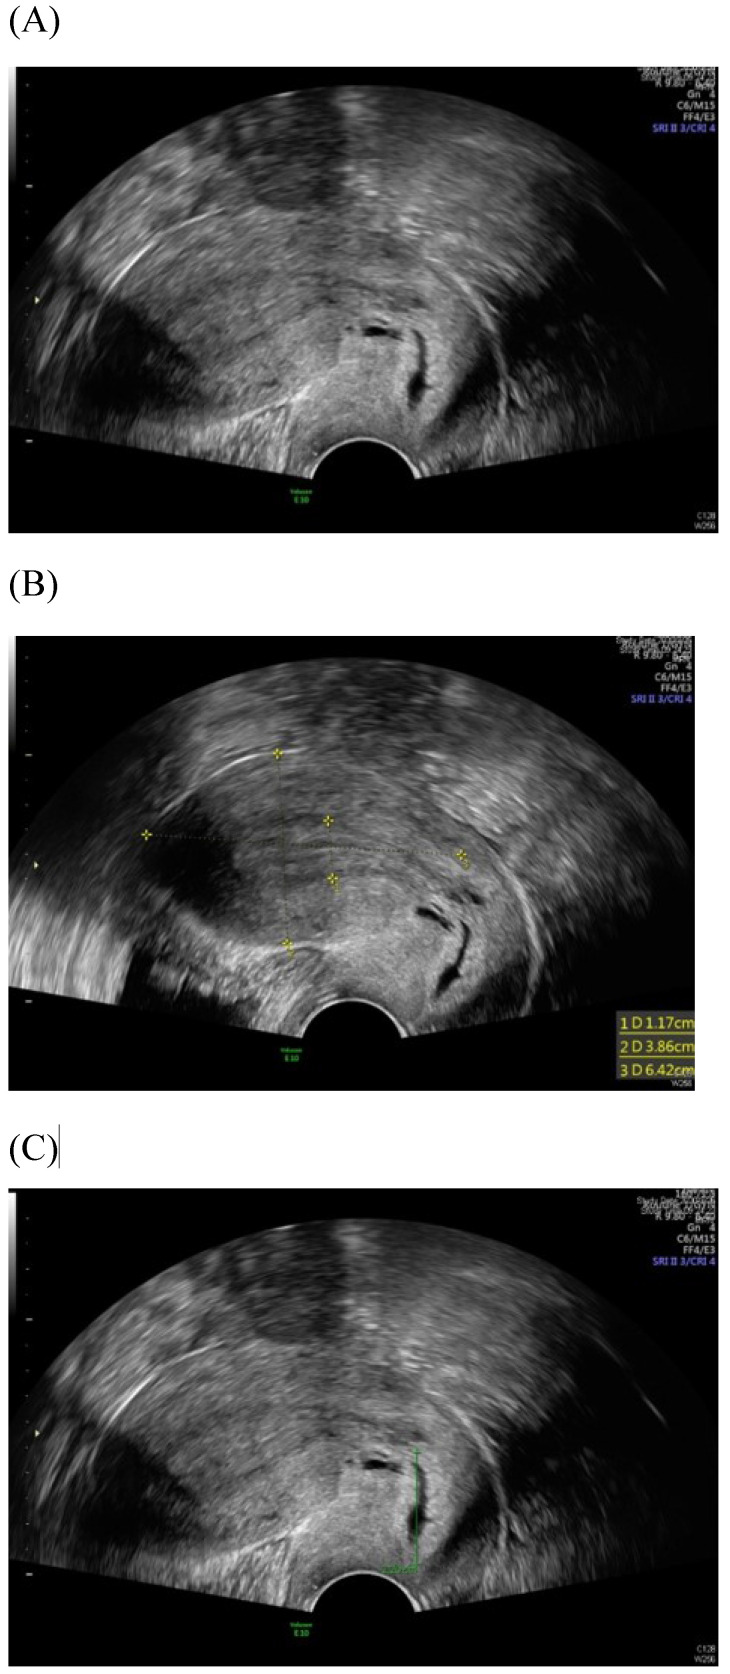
Transvaginal ultrasound image shows a longitudinal section of the uterus with normal cervix of the female aged 31-year-old. (A) Sagittal view (B) uterine size (C) cervical length.
